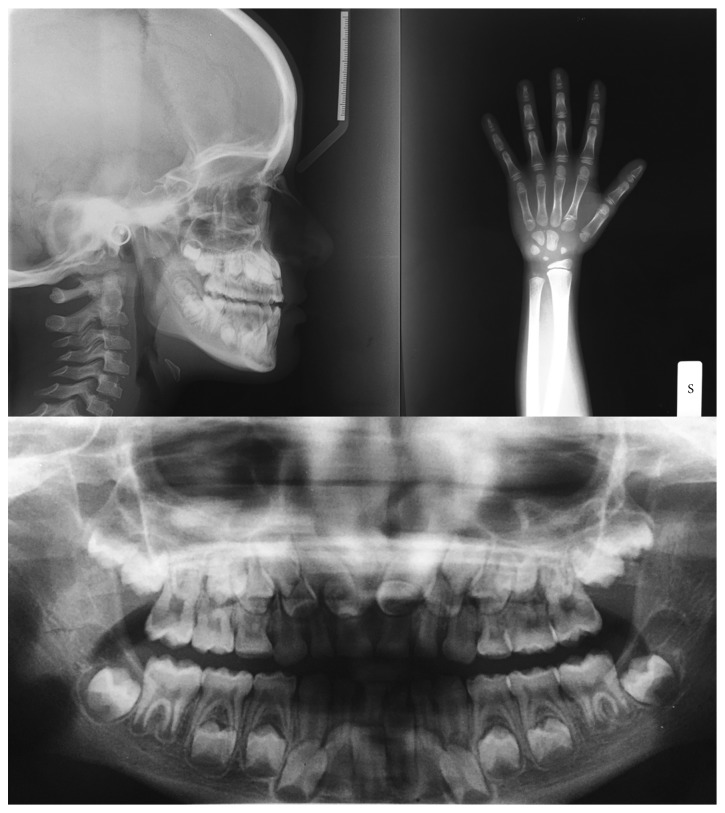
Radiographs of skull and panorex.
